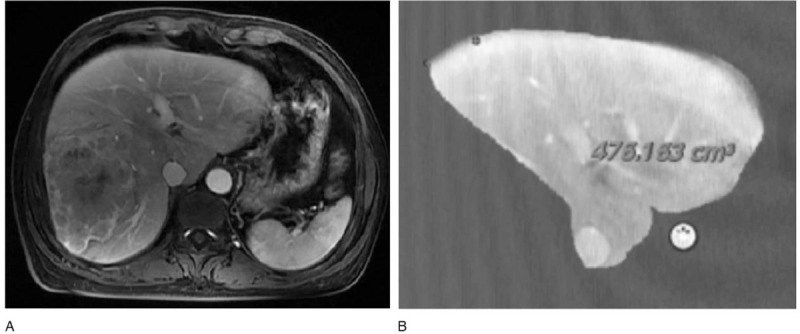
Magnetic resonance imaging scan shows (A) a huge mass in the right liver and (B) the future liver remnant after right hepatectomy.
